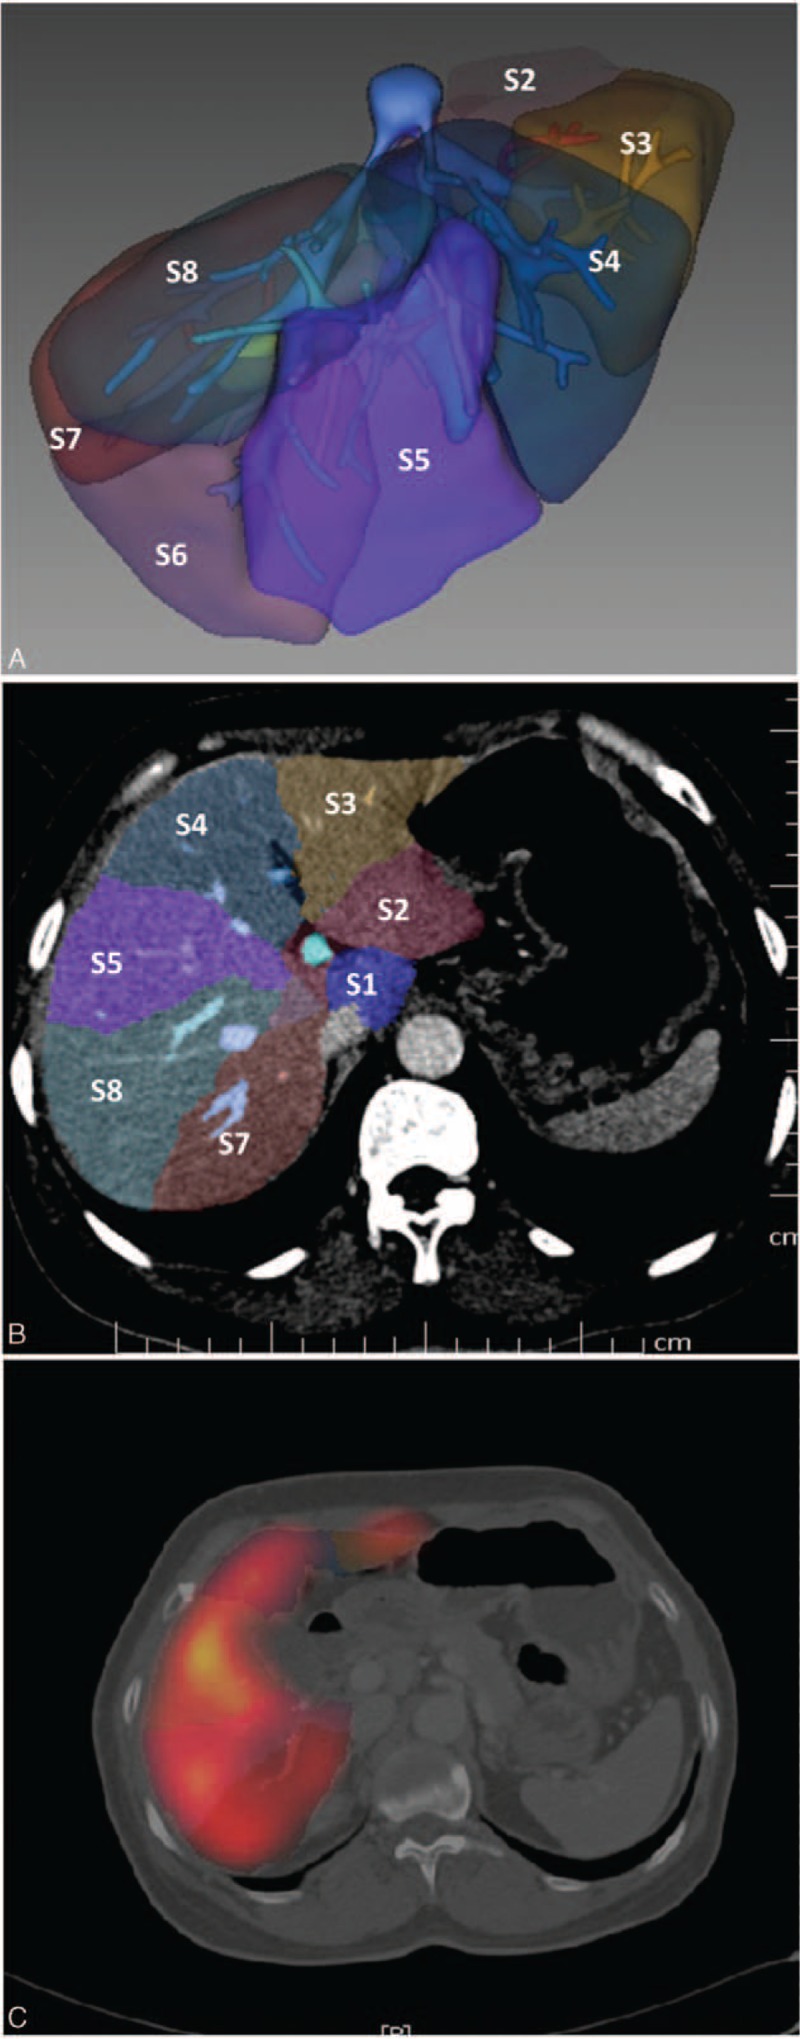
Borders of intrahepatic segments. A, Segments division according to the portal territory (3D image, S1-S8). B, Segments division according to the portal territory (transverse section image, S1-S8). C, the corresponding CT-SPECT fusion image. CT-SPECT = computed tomography–single-photon emission computed tomography.
